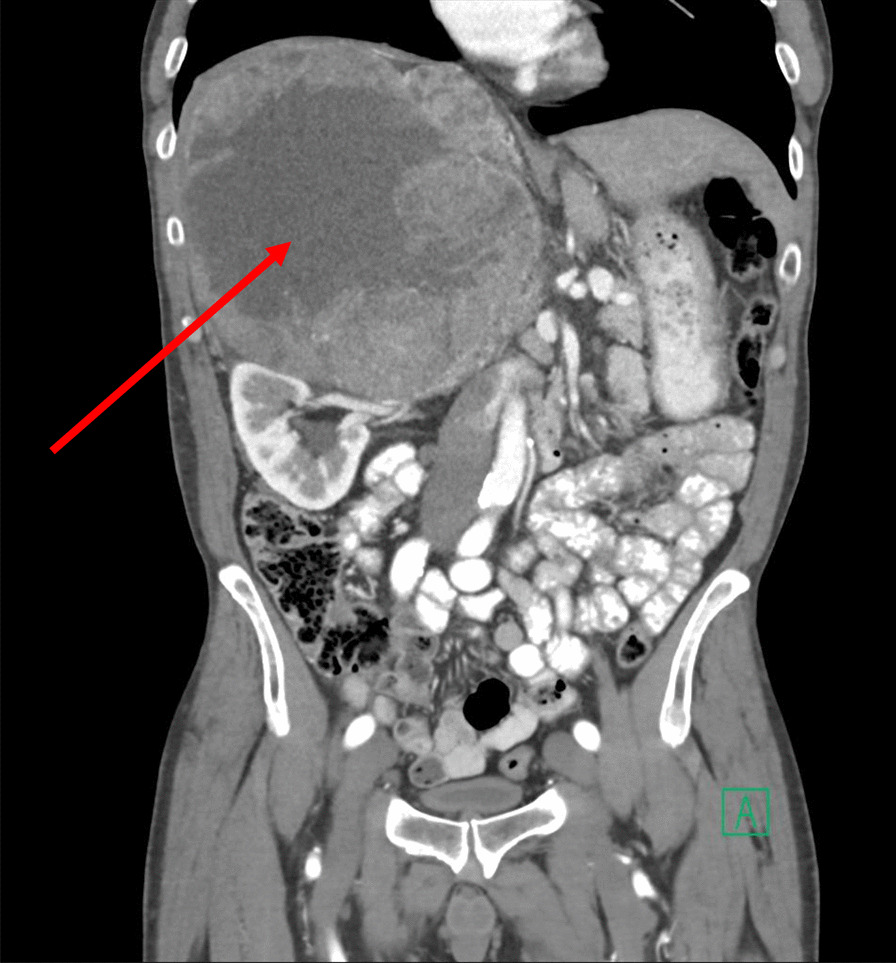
Computed tomography of the abdomen and pelvis. Arrow: adrenocortical carcinoma
